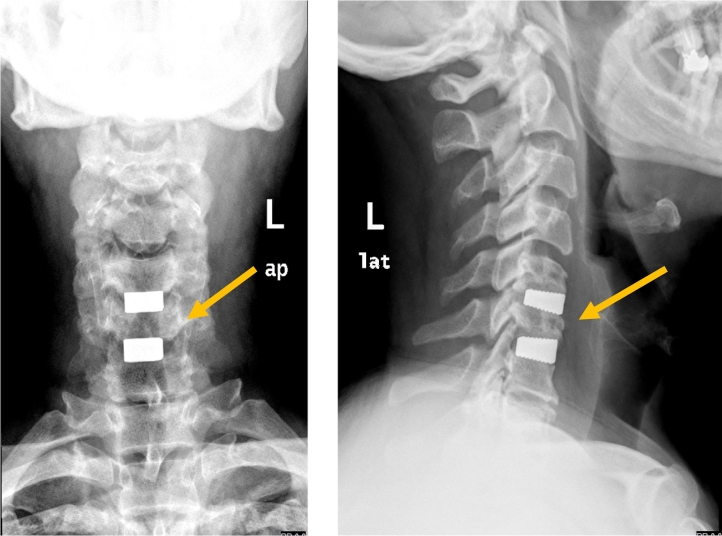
X-ray cervical spine antero-posterior and lateral views showing anterior cervical decompression and fusion at C5–C6 and C6–C7.
